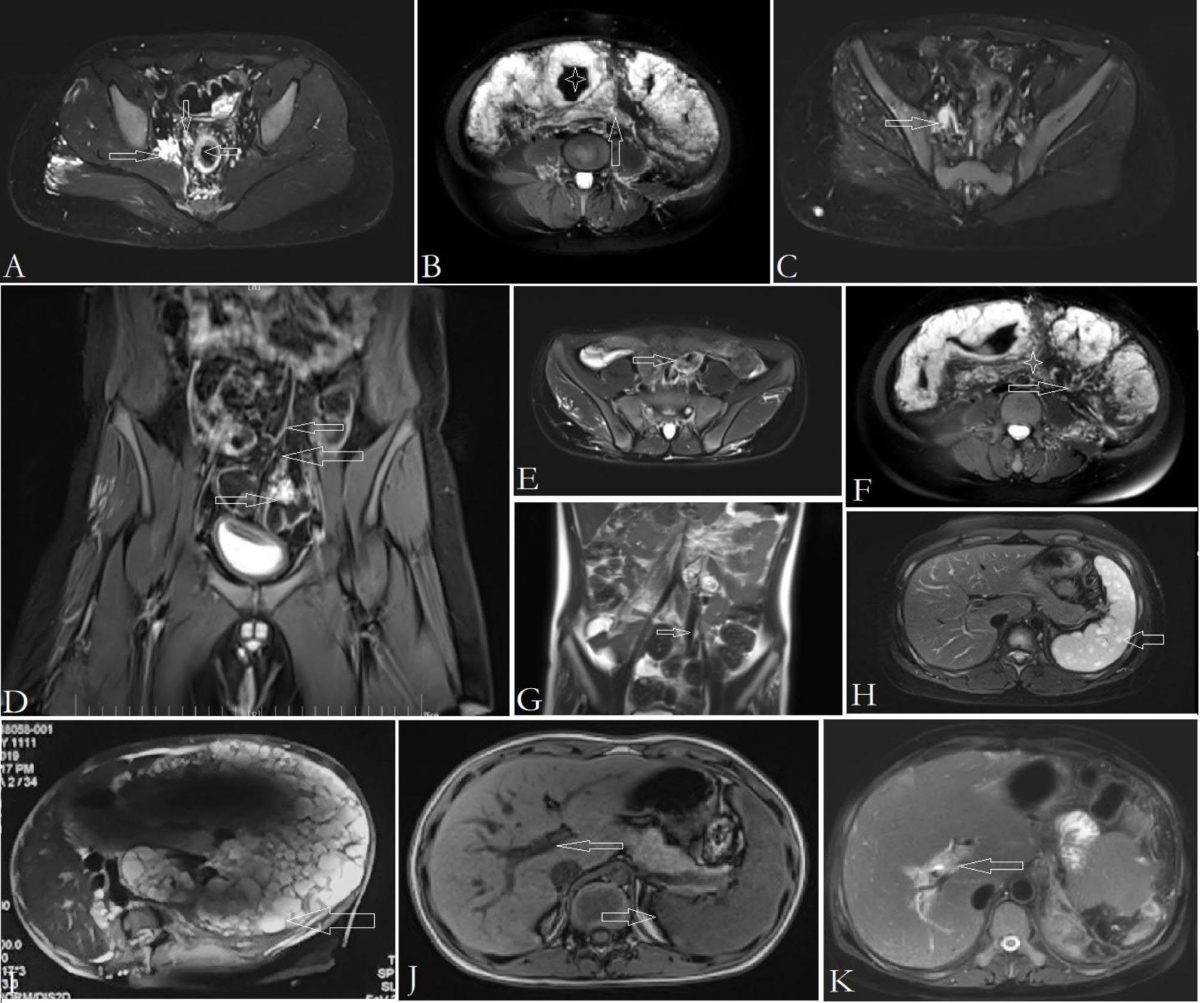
MRI findings in KTS with anorectal and anorectosigmoid VM. Panel A: Dilated, incompetent middle rectal vein (rightward arrow), perirectal VM (downward arrow), and thickening of rectal wall by VM involving (leftward arrow). Panel B: Sigmoid lumen became narrowed by thickening of the wall (asterisk). The mesentery of sigmoid colon become thickened and edematous (arrow). Panel C: Dilated, incompetent IIV (rightward arrow) is demonstrated. Typical fluid signal in the lumen is indicative of stagnation and/or reflux of blood. Panel D: Part of VM in rectosigmoid colon (rightward arrow) drains into the superior rectal vein (leftward arrows). Panel E and G: Ectatic and incompetent inferior mesenteric vein is identified (arrow). Heterogeneous signal in the lumen is indicative of blood stagnation, turbulent flow and reflux. Panel F: Dilation and tortuosity of the sigmoid vein in the mesentery (arrow). It drains into inferior mesenteric vein. Thickening and edema of the sigmoid mesentery also can be identified (asterisk). Panel H and I: Multiple cystic lymphatic malformations within the spleen (arrow). Splenectomy is indicated for massive splenomegaly (Panel I). Panel J: Main branches of portal vein become dilated (leftward arrow), and spleen enlarged (rightward arrow), which is suggestive of portal hypertension, although obstruction is not evident. Panel K: Main branches of portal vein become dilated and obstructed (arrow). Circumferential edema is notable. The spleen was resected because of portal hypertension and massive splenomegaly by lymphatic malformations
